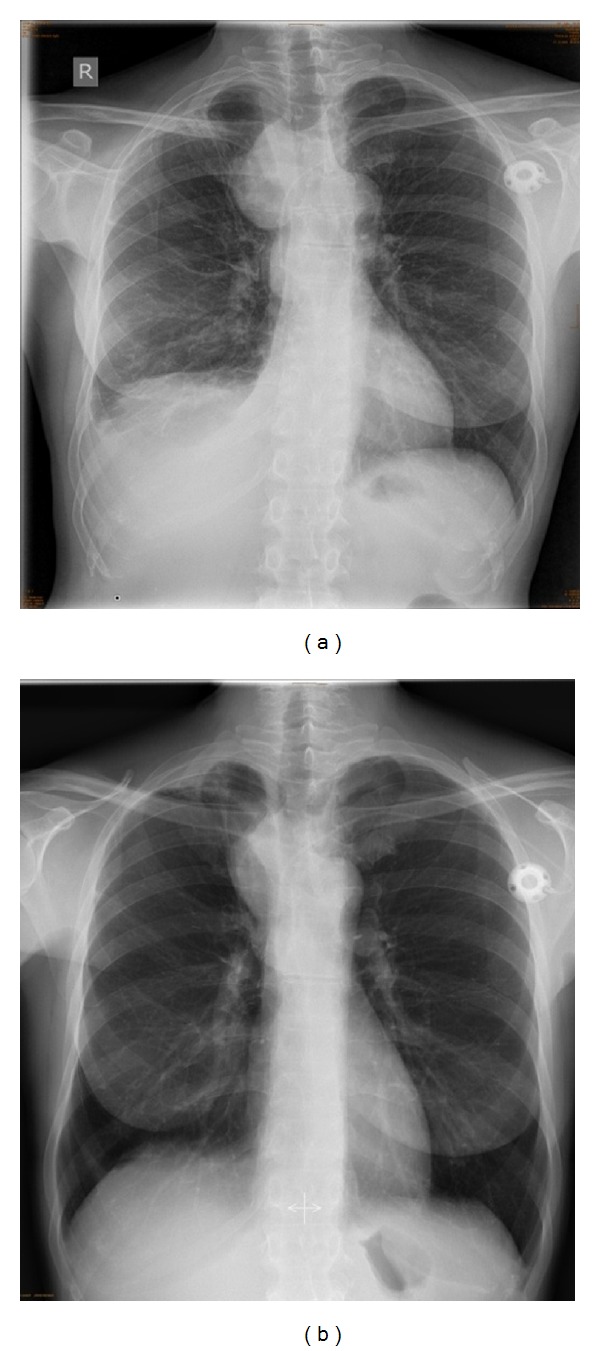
Anteroposterior chest radiograph showing a large homogenous opacity right paramediastinal and right side pleural effusion. (a) Initial presentation. (b) Response to treatment with moxifloxacin four weeks after initial presentation.
